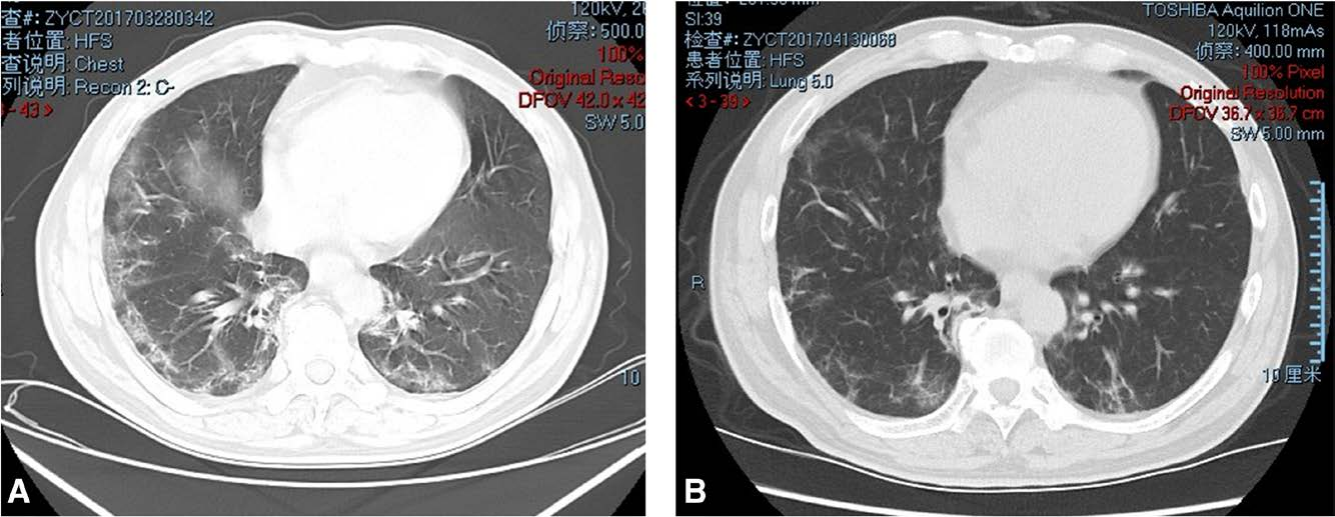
(A and B) Radiologic progression of interstitial pneumonia: (A) Diagnosis of interstitial pneumonia 2 wk following primary total knee arthroplasty; (B) chest CT scan demonstrating resolution of pneumonia during the 2nd wk of antibiotic treatment. CT = computed tomography.
